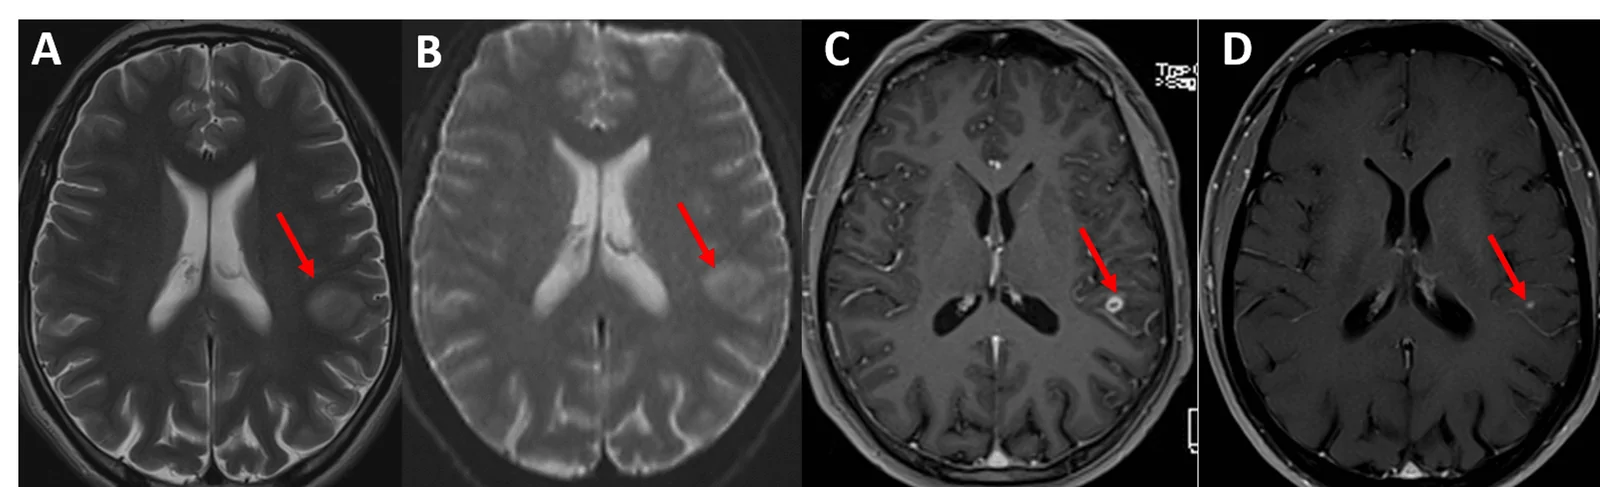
Brain magnetic resonance imaging (A) T2-weighted imaging and (B) diffusion-weighted imaging show a left parietal lesion with surrounding edema. (C) After contrast administration, the left parietal cysticercal lesion demonstrates ring enhancement. (D) Follow-up magnetic resonance imaging performed three months after treatment initiation shows the resolution of the ring enhancement after gadolinium administration.
